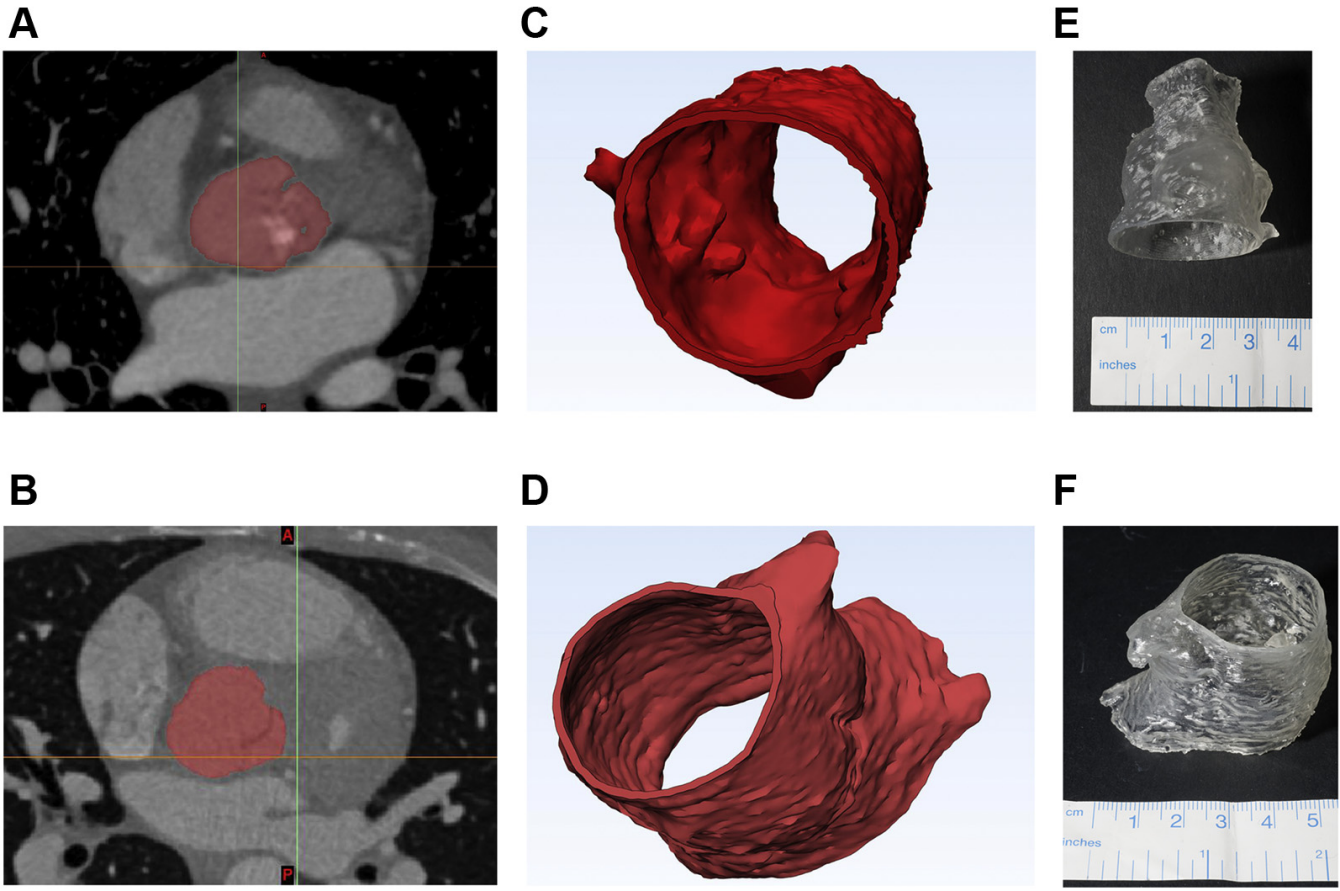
Patient-Specific Models of the Aortic Roots Were Derived From Clinically Indicated Computed Tomography Datasets (A, B) Segmentation was carried out by using Materialise (Leuven, Belgium) Mimics software to select the area of interest (highlighted in red)—in this case, the aortic root. (C, D) Materialize 3-matic was used to reconstruct the aortic roots in 3 dimensions (3D) and produce an stereolithography file suitable for importing into the 3D printer software. (E, F) The aortic roots were then printed in a flexible resin. Images for Patients #1 and #2 displayed are in the top and bottom rows, respectively.
